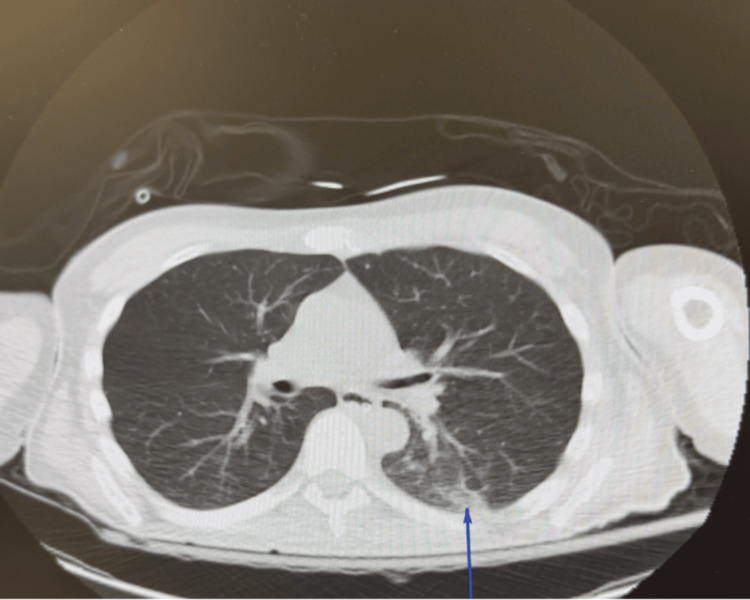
Computed tomography of the chest showing patchy consolidation in the posterior left lower pulmonary lobe (blue arrow).
